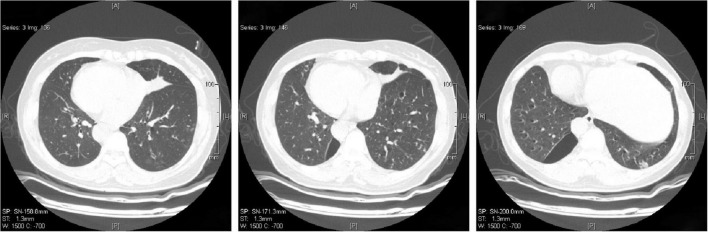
Chest computed tomography shows inflammation in both sides of lung lobes.
